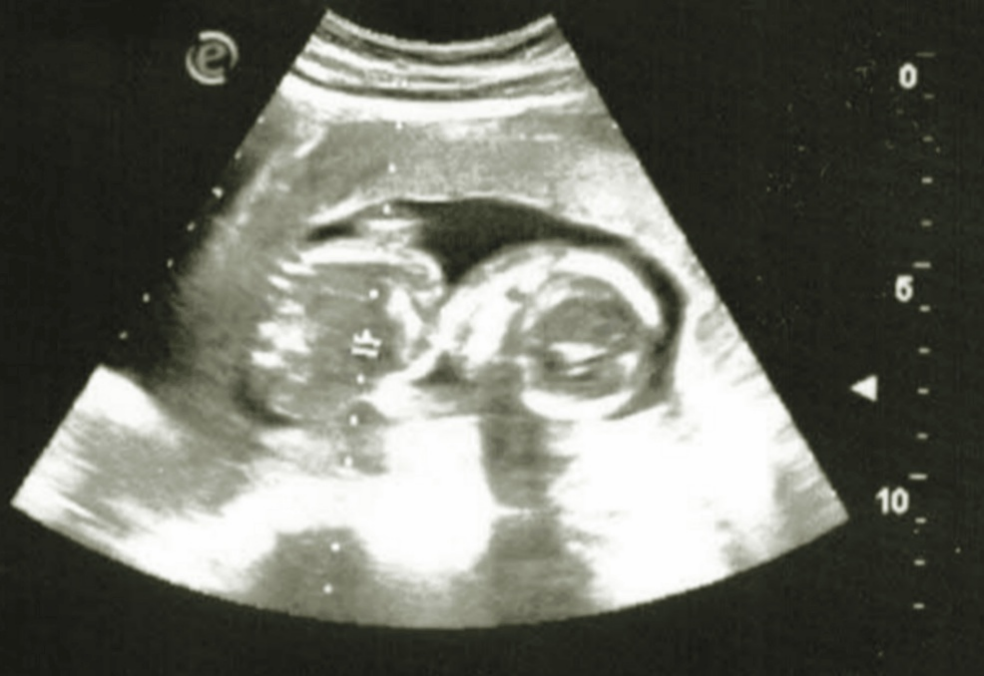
Ultrasound picture of the AC: 129.9 mm (reference limits: 104-144 mm) AC, abdominal circumference
